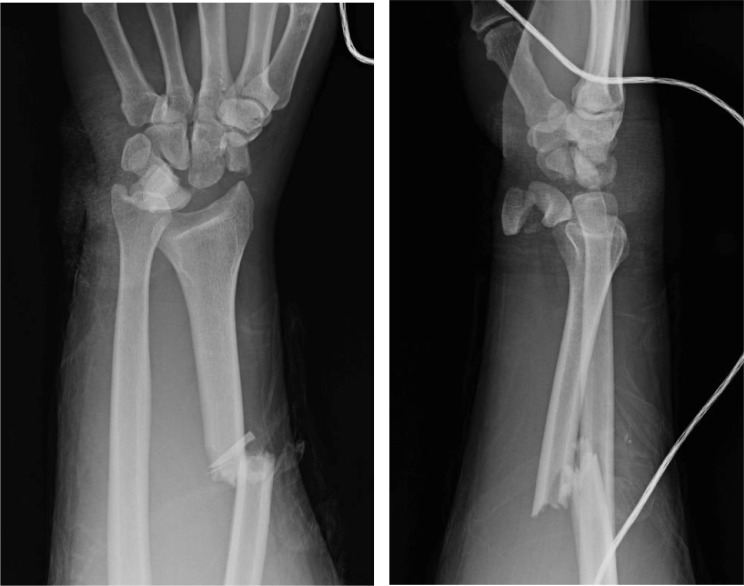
Patient 2: Initial radiographs of the right wrist demonstrate trans-capitate trans-scaphoid lunate dislocation as well as Galeazzi type fracture-dislocation including positive ulnar variance.
